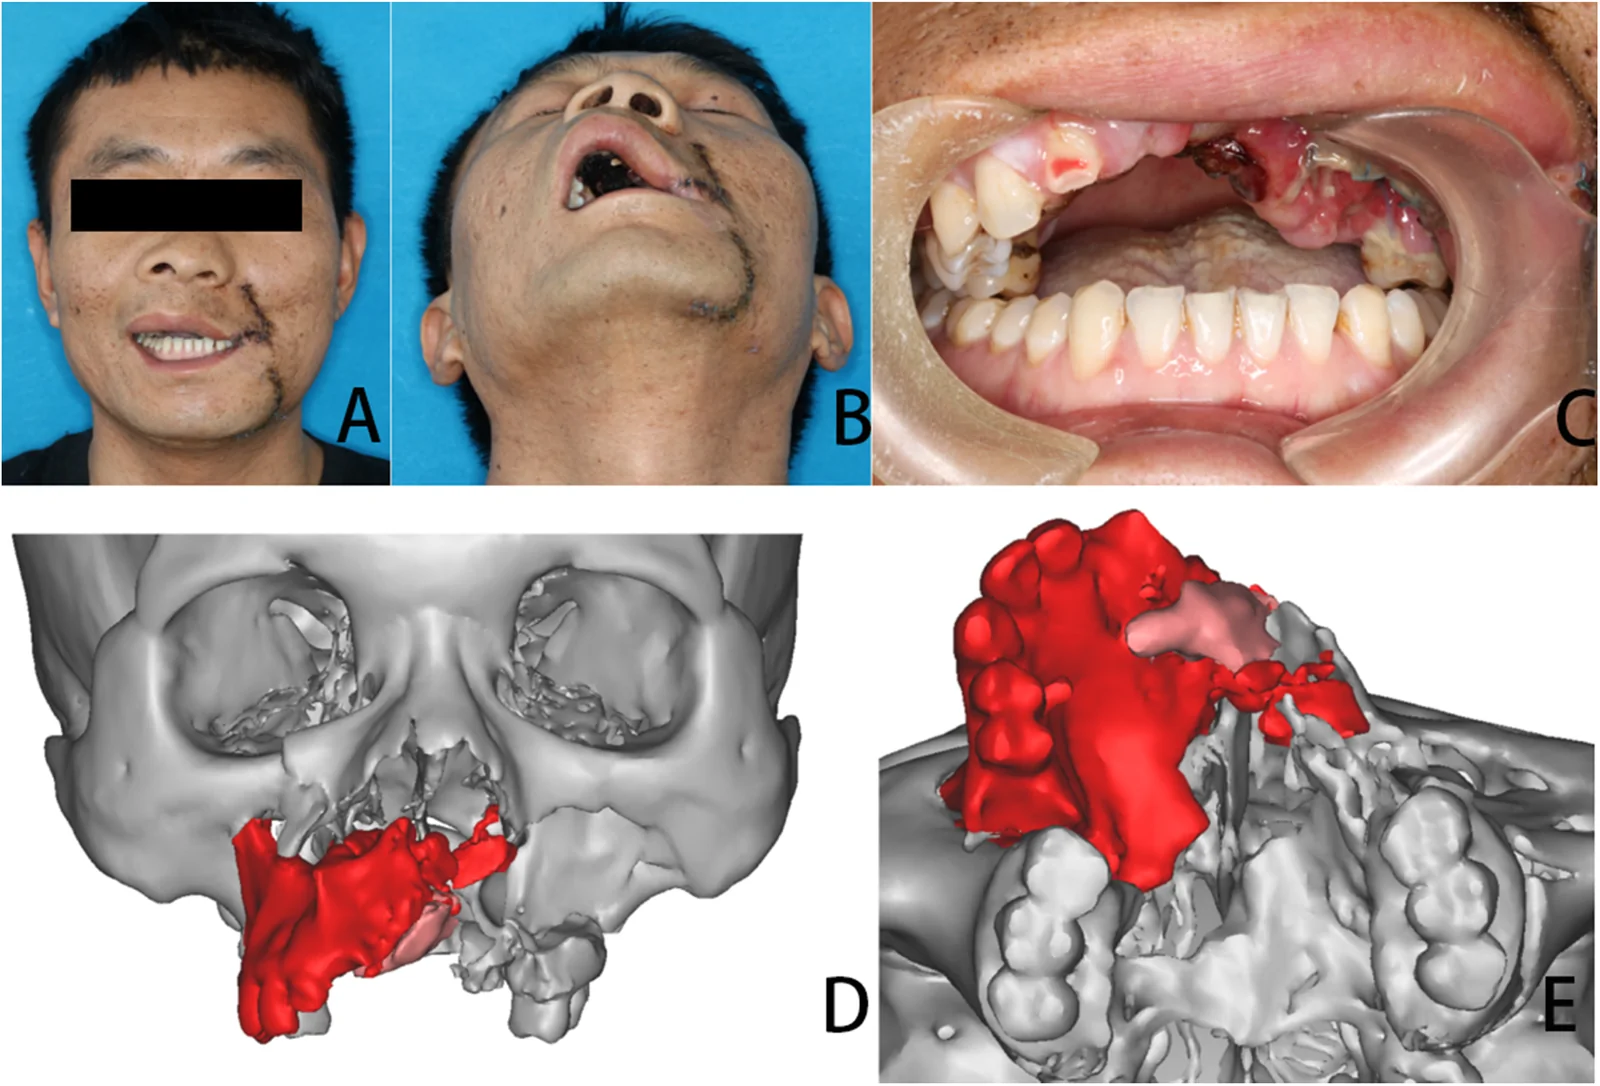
Preoperative clinical and radiographic images of case 1. (A,B) Extraoral photographs. (C) Intraoral photograph. (D,E) Three-dimensional CT reconstructions.
